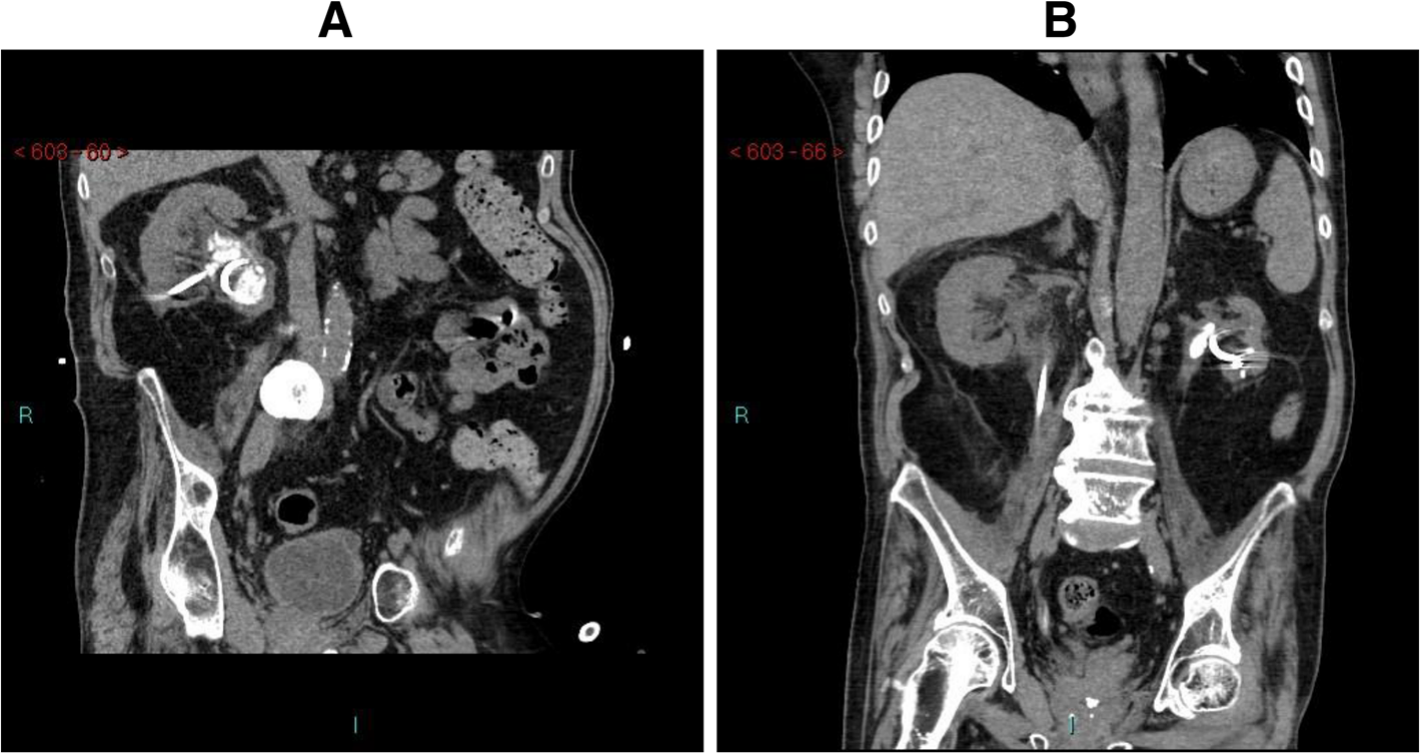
Case 3: Computed Tomography of kidneys, coronal view. (A) right kidney: nephrostomy in place; several calculi in renal pelvis, and calcification in aorta. (B) stent in right ureter; nephrostomy in left kidney; stone in left renal pelvis; and left kidney is atrophic.
